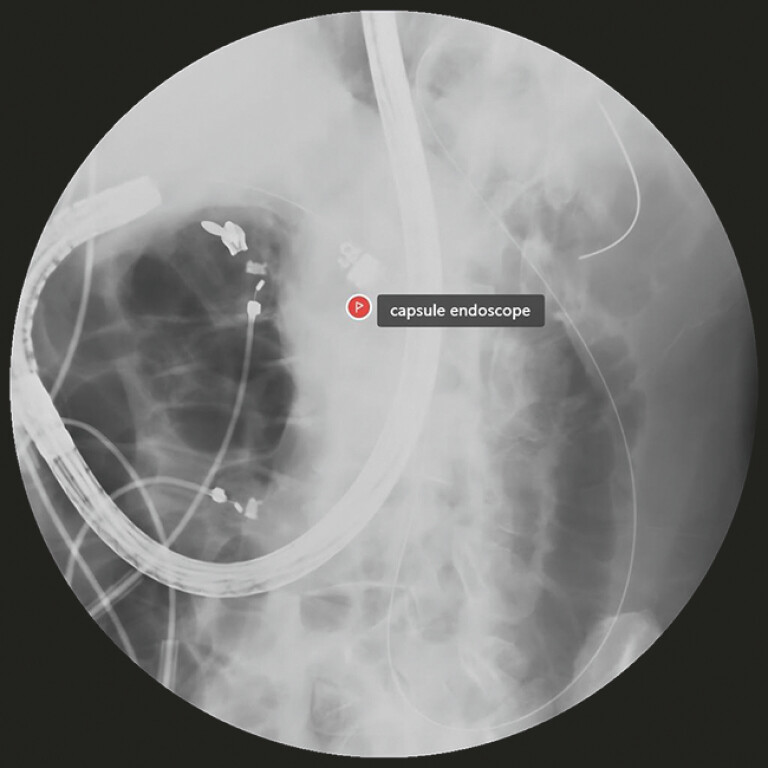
The capsule endoscope was successfully placed in the duodenum under X-ray surveillance.
